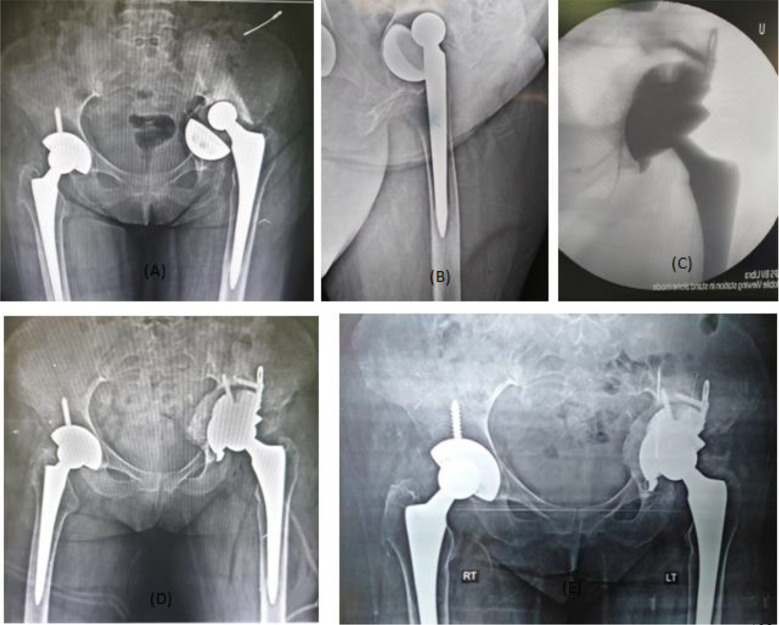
Male 50 years old with type IIIB defect treated with Lima TT revision shell and allograft. (A) Showing the preoperative X-ray AP view, (B) preoperative X-ray lateral view, (C) intraoperative image intensifier radiographs after cup application, (D) postoperative X-ray and (E) X-ray after 2 years shows allograft was completely incorporated with the host bone with stable reconstruction.
